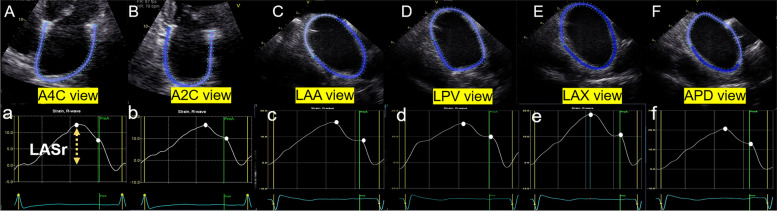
LA reservoir strain measurements. A LASr derived from the A4C view of TTE; (B) LASr derived from the A2C view of TTE; (C) LASr derived from the LAA view of ICE; (D) LASr derived from the LPV view of ICE; (E) LASr derived from the LAX view of ICE; (F) LASr derived from the APD view of ICE. A4C, apical 4-chamber view; A2C, apical 2-chamber view; LASr, left atrial reservoir strain; TTE, transthoracic echocardiography
